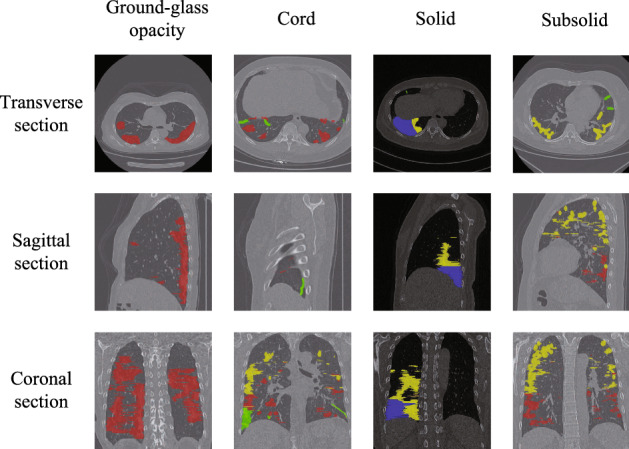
Typical four lesion subtypes in CT images of COVID-19. The labels and regions are given by medical experts. The red area represents ground-glass opacity, the green area represents cord, the blue area represents solid and the yellow area represents subsolid
